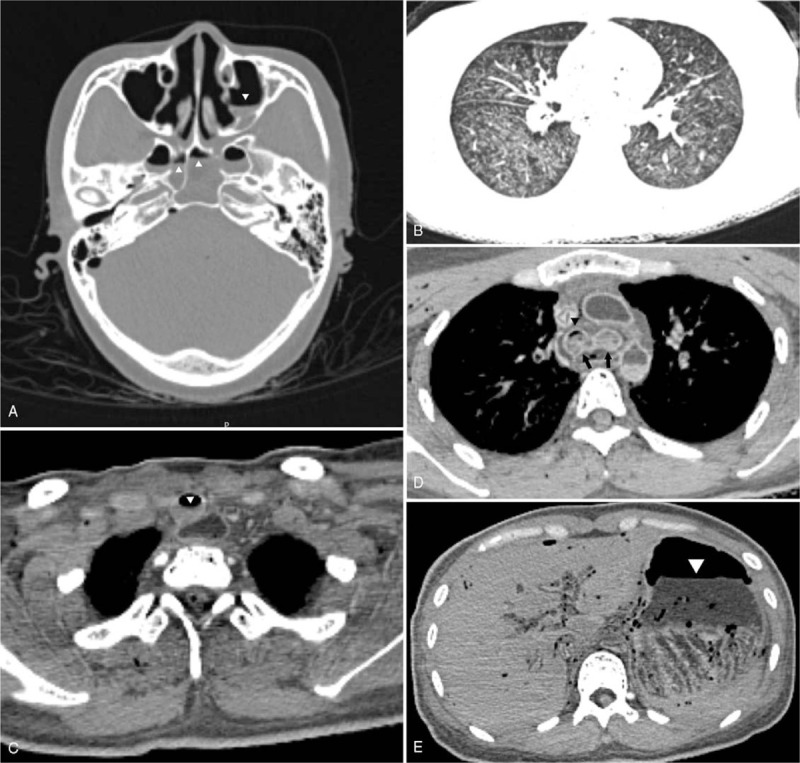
Postmortem computed tomography shows (A) air/fluid in the sphenoidal sinus (arrowheads) and the left maxillary sinus (arrowhead); (B) mosaic patterns of the lungs; (C, D) air/fluid in the trachea (arrowhead) and fluid in the main bronchi (arrows) with an air/fluid level on the right side (arrowhead); and (E) air/fluid in a distended stomach (arrowhead).
